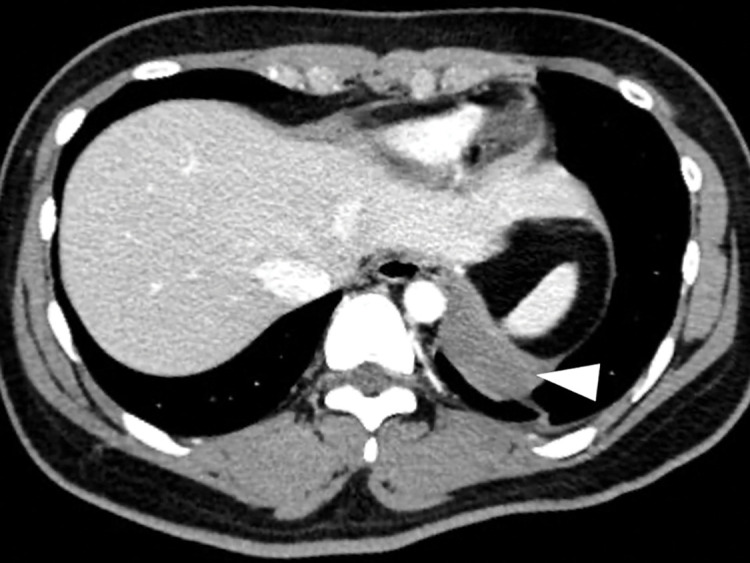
A chest CT showed a well-circumscribed mass (arrowhead) along the left diaphragm.
